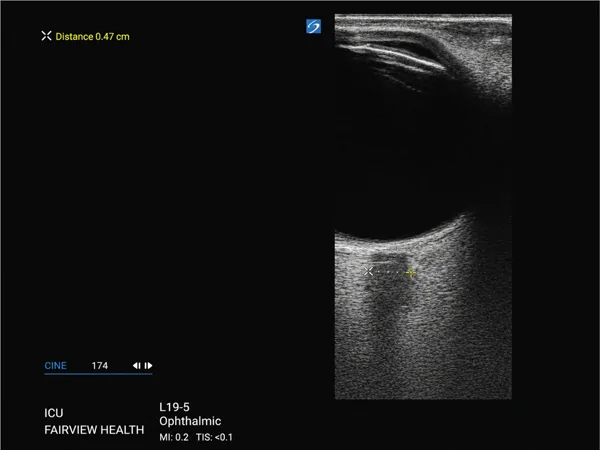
Bedside ocular ultrasonography demonstrating an optic nerve sheath diameter of 0.47 cm within normal limits and not suggestive of elevated intracranial pressure. AI was used in an attempt to decrease glare and increase image quality.
